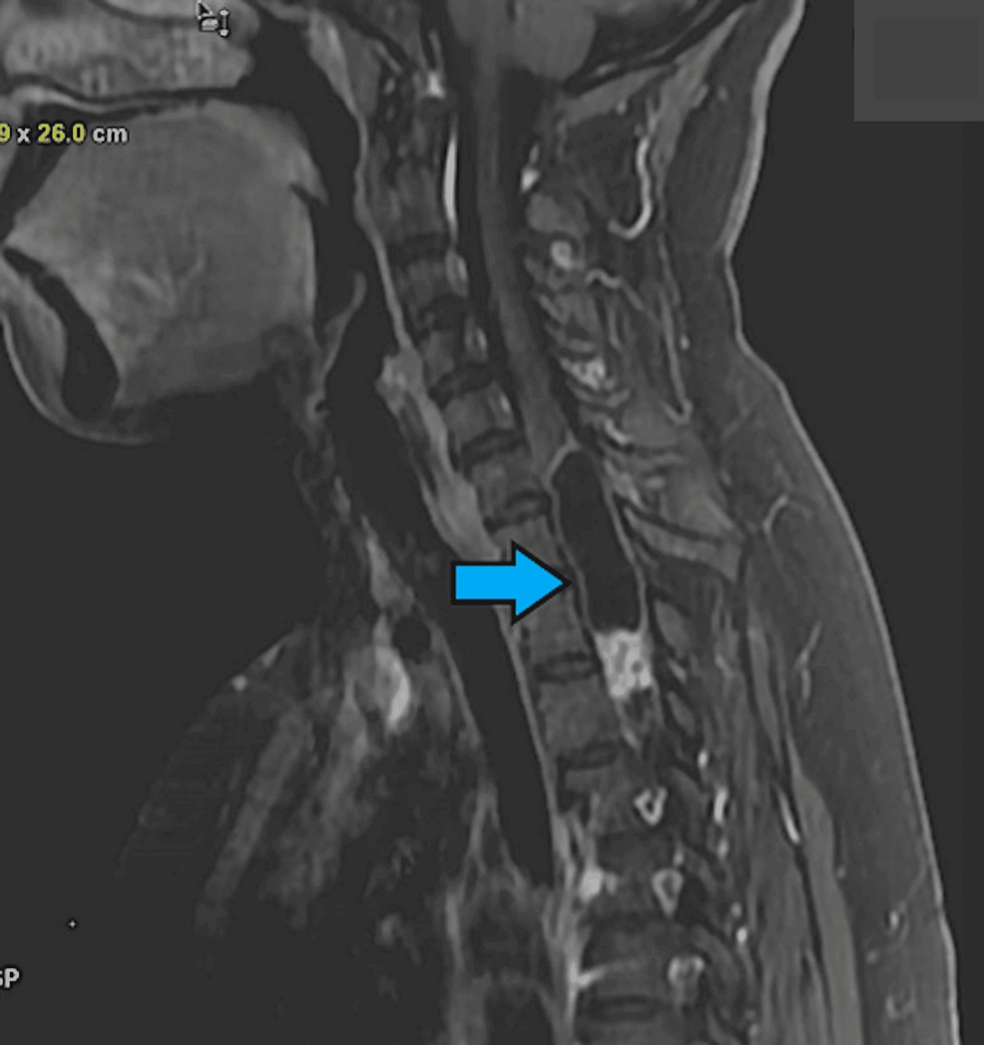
Post-contrast MRI depicting an elongated cystic lesion with a solid component at the C7-D4 level of the spinal cord (blue arrow). MRI: magnetic resonance imaging
